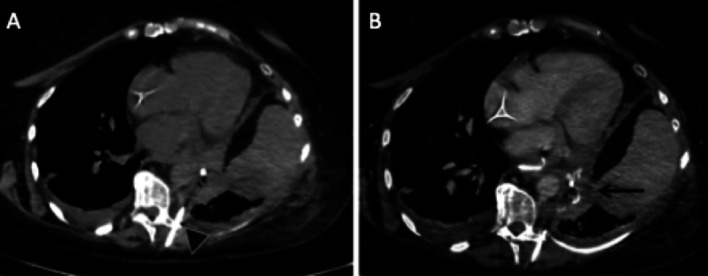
Oesophagopleural fistula. Axial CT images (mediastinal window) without injection and without ingestion of iodinated contrast medium (A), with injection and ingestion of iodinated contrast medium (B). We note the absence of spontaneous hyperdensity of the pleural empyema, and its filling with iodinated contrast medium after ingestion (black arrow). A drain was already in the empyema at the time of CT acquisition (black arrowhead)L.
